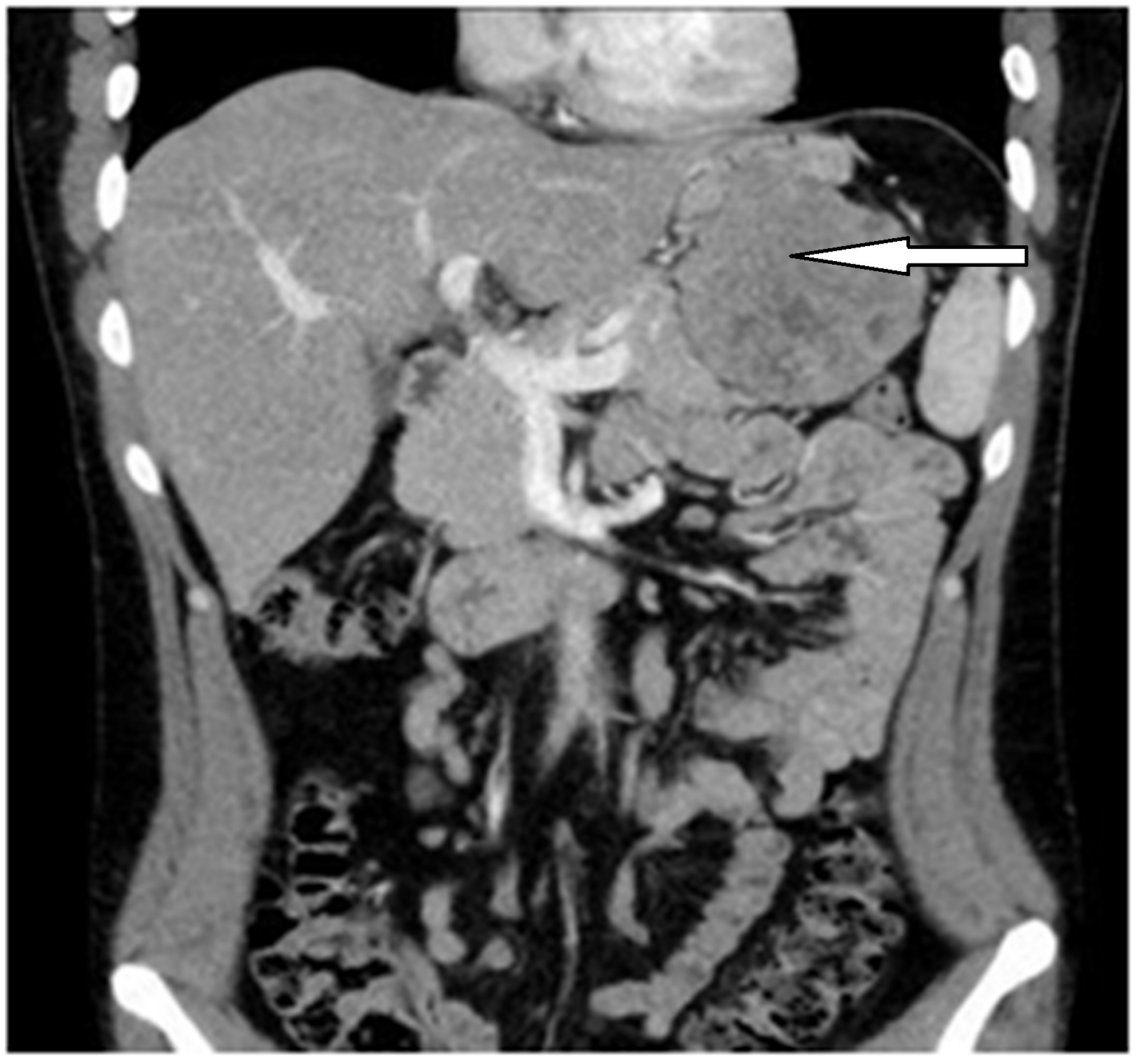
Abdominal CT scan image (coronary view) showing the mass arising from the proximal gastric greater curvature of the stomach displacing the tail pancreas without evidences of pancreatic invasion or hepatic metastasis.
